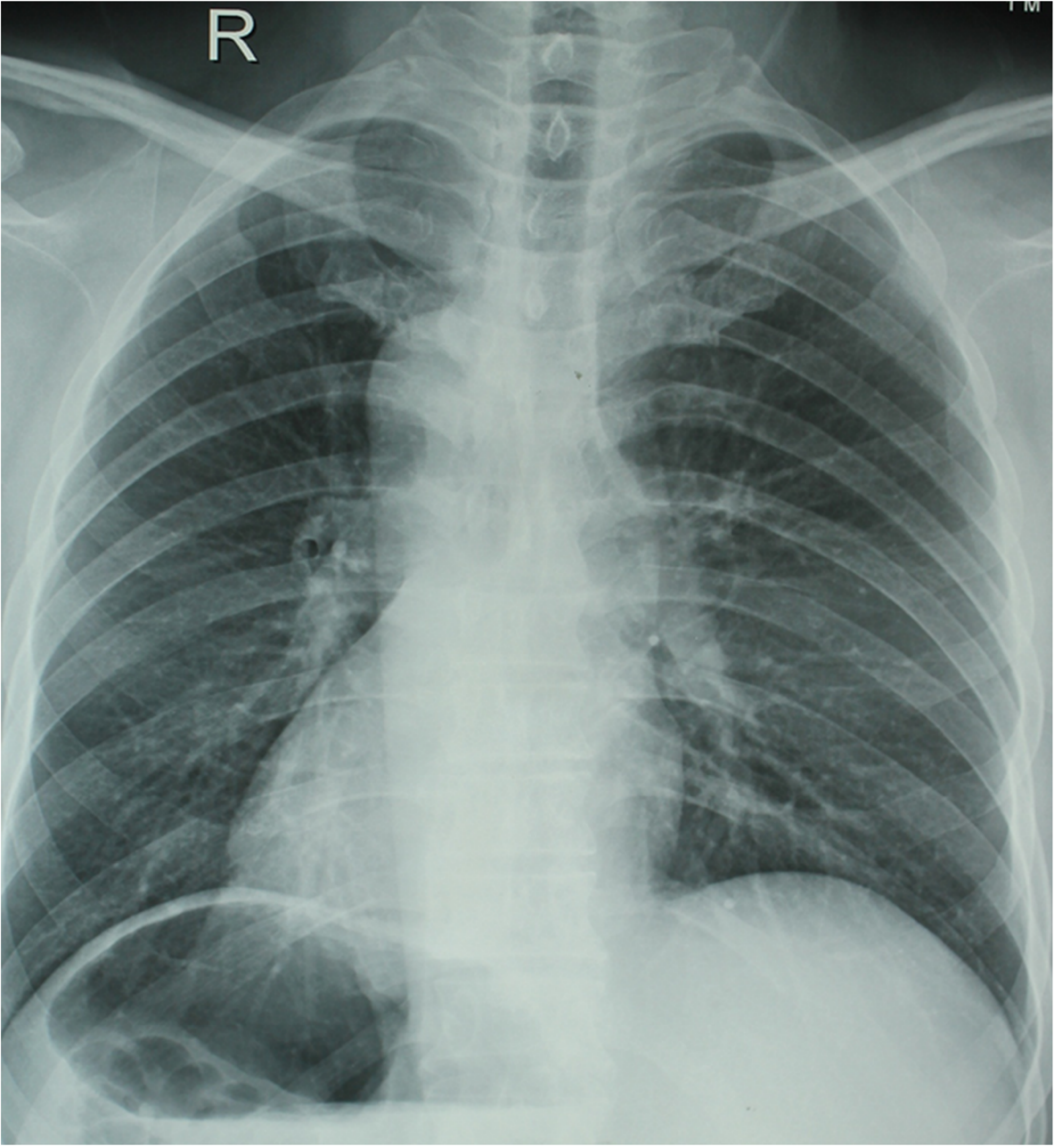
Chest X-ray demonstrating dextrocardia.
